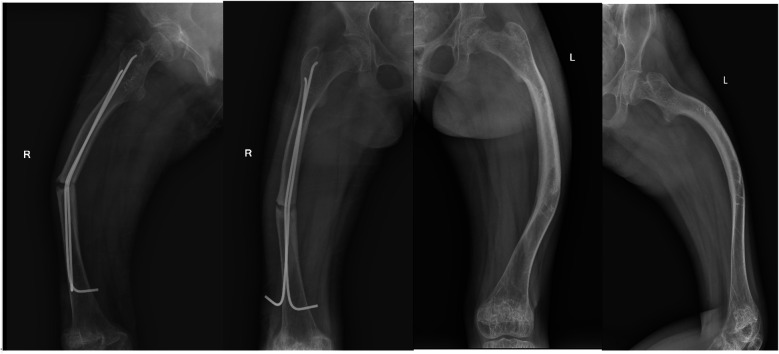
X-ray showing right femoral deformity, left femoral deformity, and remnants of elastic intramedullary nails in the marrow cavity of the right femur.
